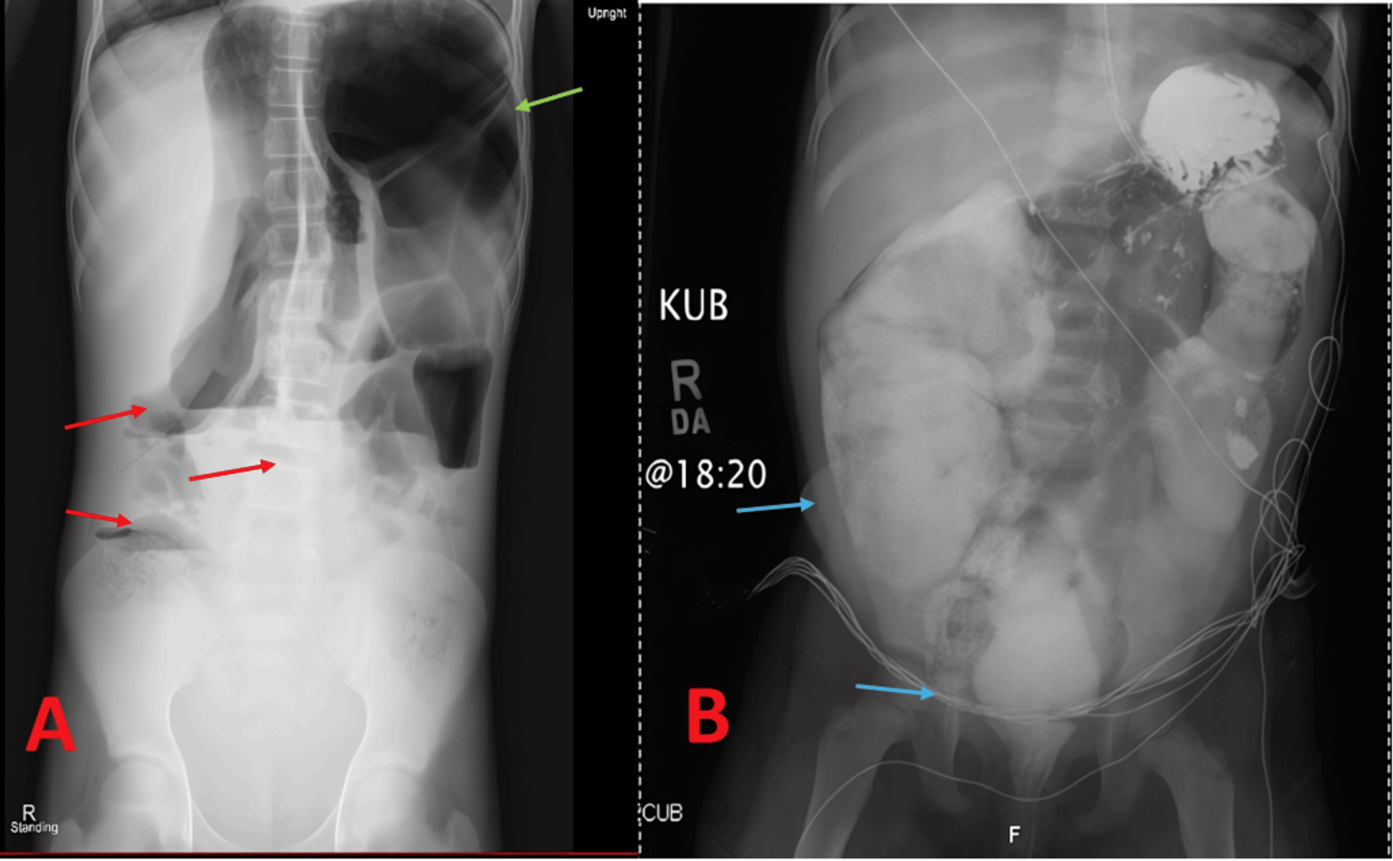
Final Follow-up and Recovery (A) Day 14 upright abdominal radiograph revealing normalization of the bowel gas pattern. Green arrows highlight mildly dilated bowel loops, and red arrows indicate air-fluid levels. (B) Abdominal KUB with lines (blue arrow) demonstrating contrast passing fully through the bowel and reaching the rectum, confirming patency and absence of mechanical obstruction. Minimal residual distension is observed, and the supportive lines remain in place prior to removal.
